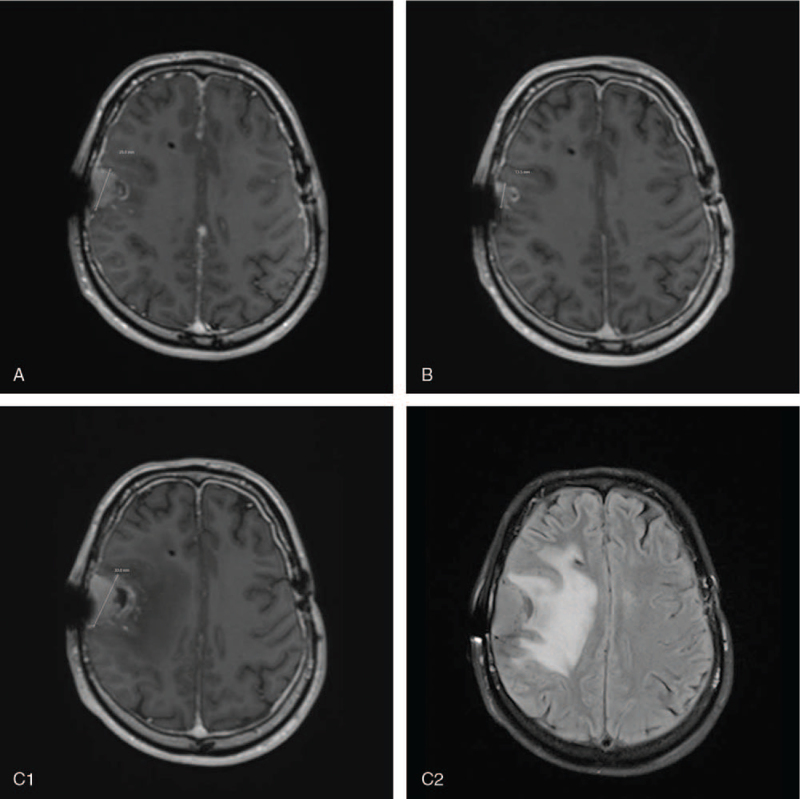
Axial post contrast T1-weighted magnetic resonance imaging showing enhanced extra-axial lesion in the region of right parietal lobe (A–C1). A and B show post chemotherapy tumor size regression. C1 shows the axial T1-w MRI tumor size progression, with a diffuse hyperintense area in the right hemisphere denoting extensive edema, as shown on axial T2 - weighted (TIRM) magnetic resonance imaging (C2).
